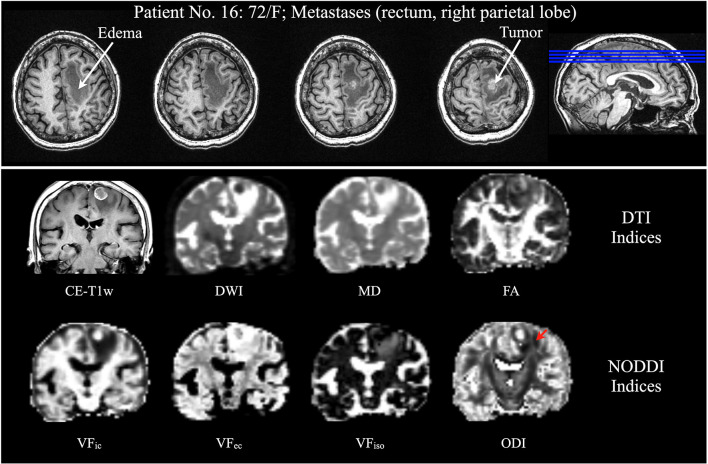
Patient No. 16, a 72-year-old woman with rectum metastasis in the right parietal lobe. (Top) Axial view of CE-T1WI displays an enhancing tumor in the right superior frontal lobe, with significant vasogenic edema. (Bottom) Coronal view of CE-T1WI, DWI, DTI indices (MD, and FA), and NODDI indices (VF_*ic*_, VF_*ec*_, and VF_*iso*_) could not reveal the CST except the ODI from NODDI (red arrow). CE-T1WI, contrast enhanced T1-weighted images; DWI, diffusion-weighted imaging; DTI, diffusion tensor imaging; MD, mean diffusivity; FA, fractional anisotropy; NODDI, neurite orientation dispersion and density imaging; VF_*ic*_, intracellular volume fraction; VF_*ec*_, extracellular volume fraction; and VF_*iso*_, isotropic volume fraction; CST, corticospinal tract; and ODI, orientation dispersion index.
